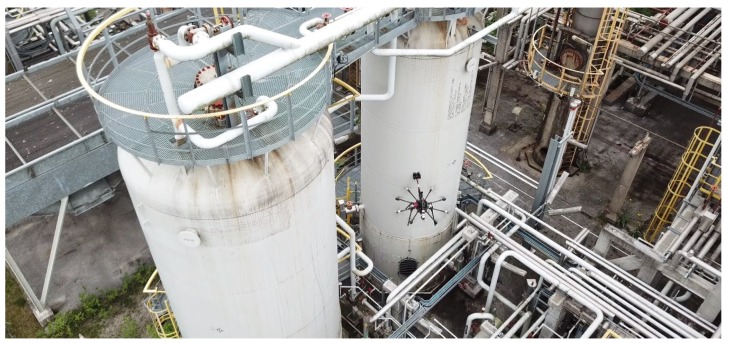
AeroX performing an ultrasonic thickness measurement in a refinery located in Germany in 18 July. The exact location cannot be disclosed due to confidentiality issues.
